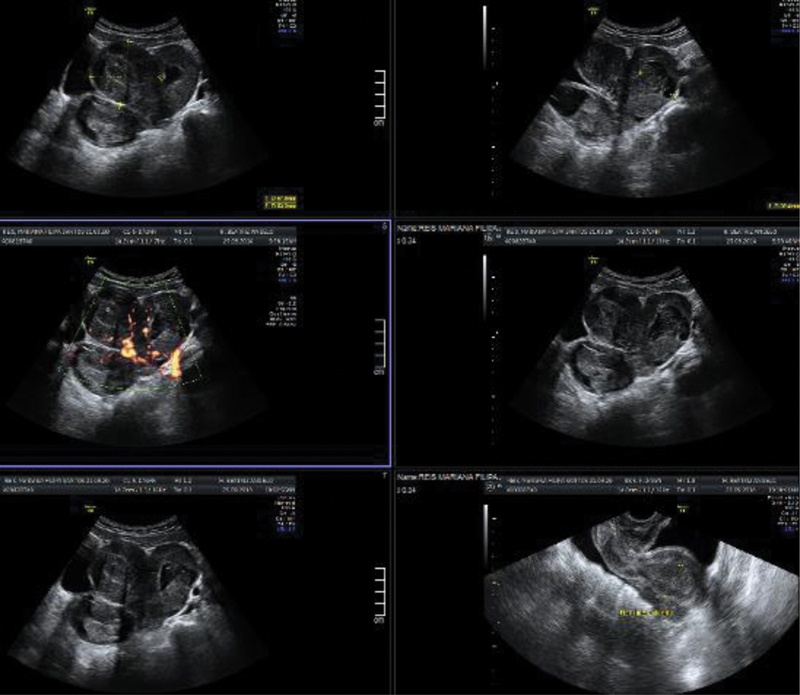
Abdominal and rectal ultrasound scan showing “bicorporal uterus, with a right hemicavidity with irregular endometrial thickening”.
